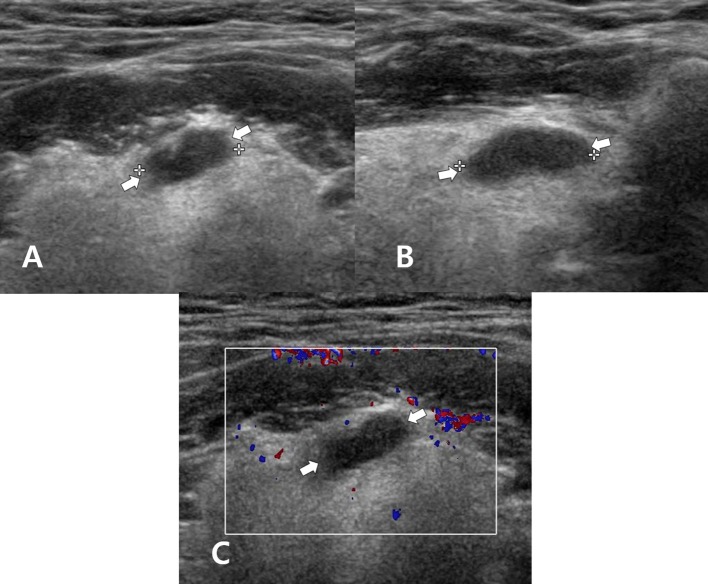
A 60~65-year-old man who underwent total thyroidectomy and one session of radioactive iodine ablation (160 mCi) for papillary thyroid carcinoma. On the follow-up neck ultrasonography (US), transverse gray-scale (A), longitudinal gray-scale (B), and transverse color Doppler (C) US images show a well-defined cystic lesion with a flat-to-ovoid shape in the suprahyoid, anterior upper midline neck (arrows, 1.3 cm in length).
